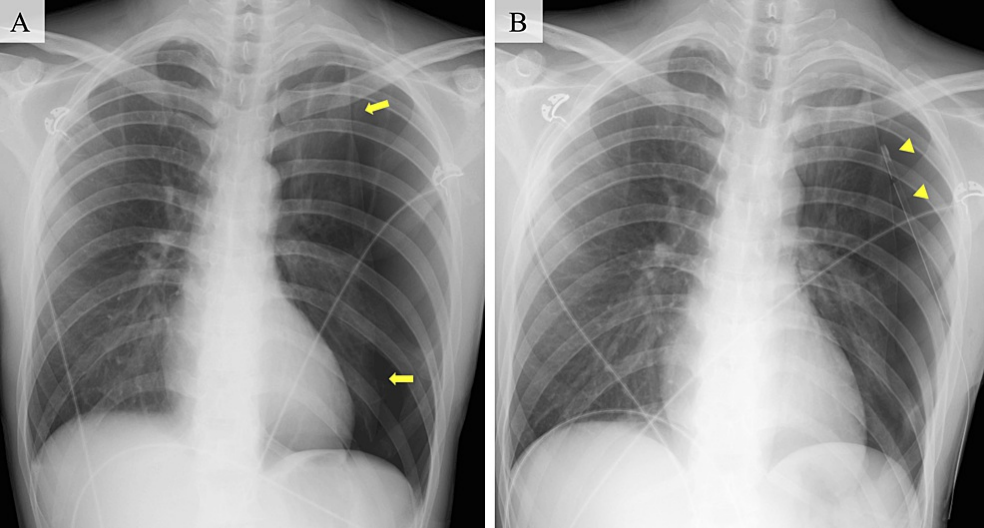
Chest radiography (A) An AP chest radiograph showing a collapsed left lung without pleural effusion (yellow arrow). (B) An AP chest radiograph showing an inserted chest tube in the left thorax (yellow arrowhead). AP: anterior-posterior
